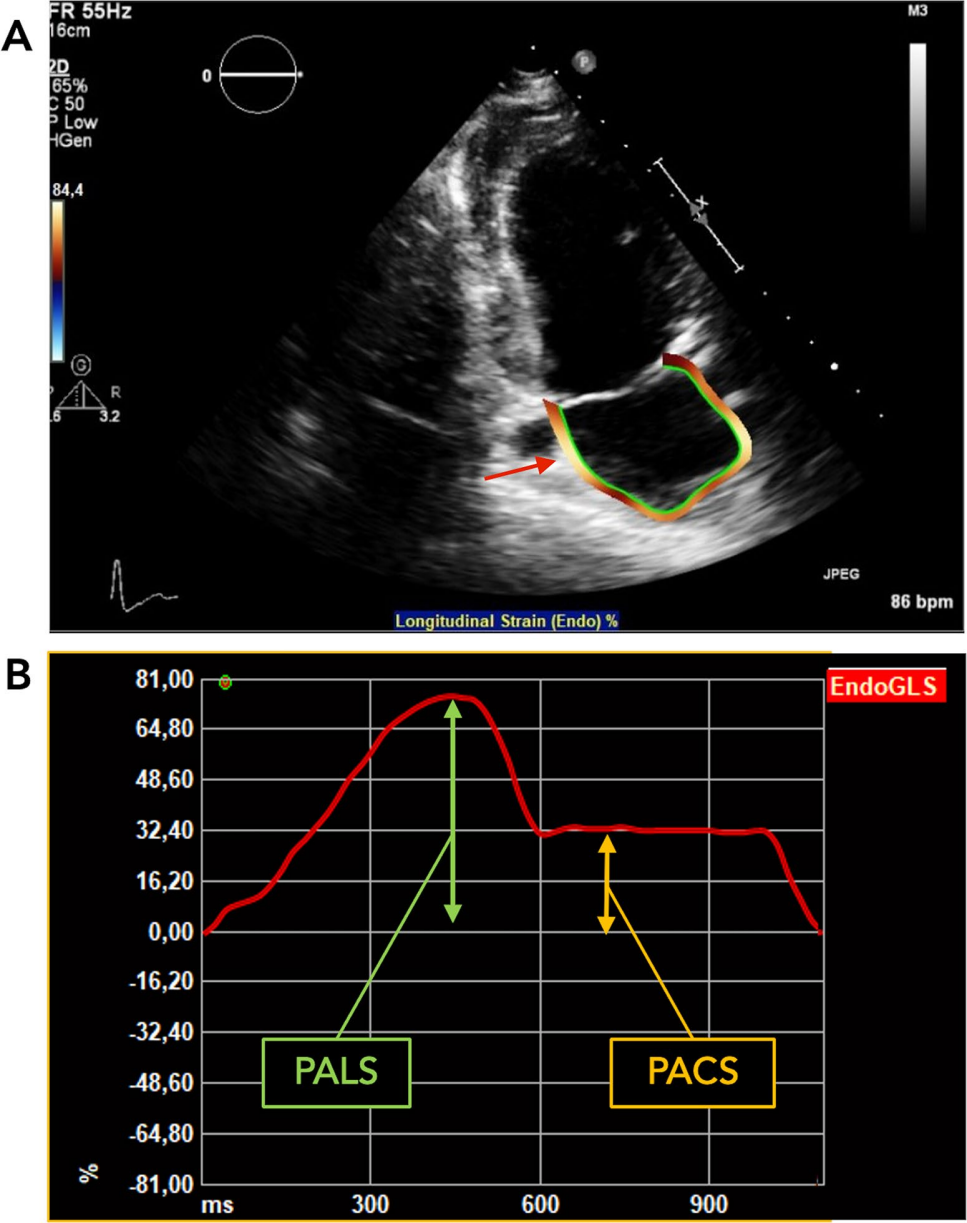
This figure shows left atrial strain analysis using speckle tracking echocardiography in 2-chamber view. (A) End-systolic automatically selected left atrial contour (red arrow). (B) LA strain profile (red line) of an ACHD patient using speckle tracking echocardiography during a complete cardiac cycle (end‑diastole to end‑diastole or R-R gated). PALS was calculated as the difference between peak and minimal strain values (green arrows). PACS represents the absolute value of the difference between minimal and plateau-phase strain values (yellow arrows)
